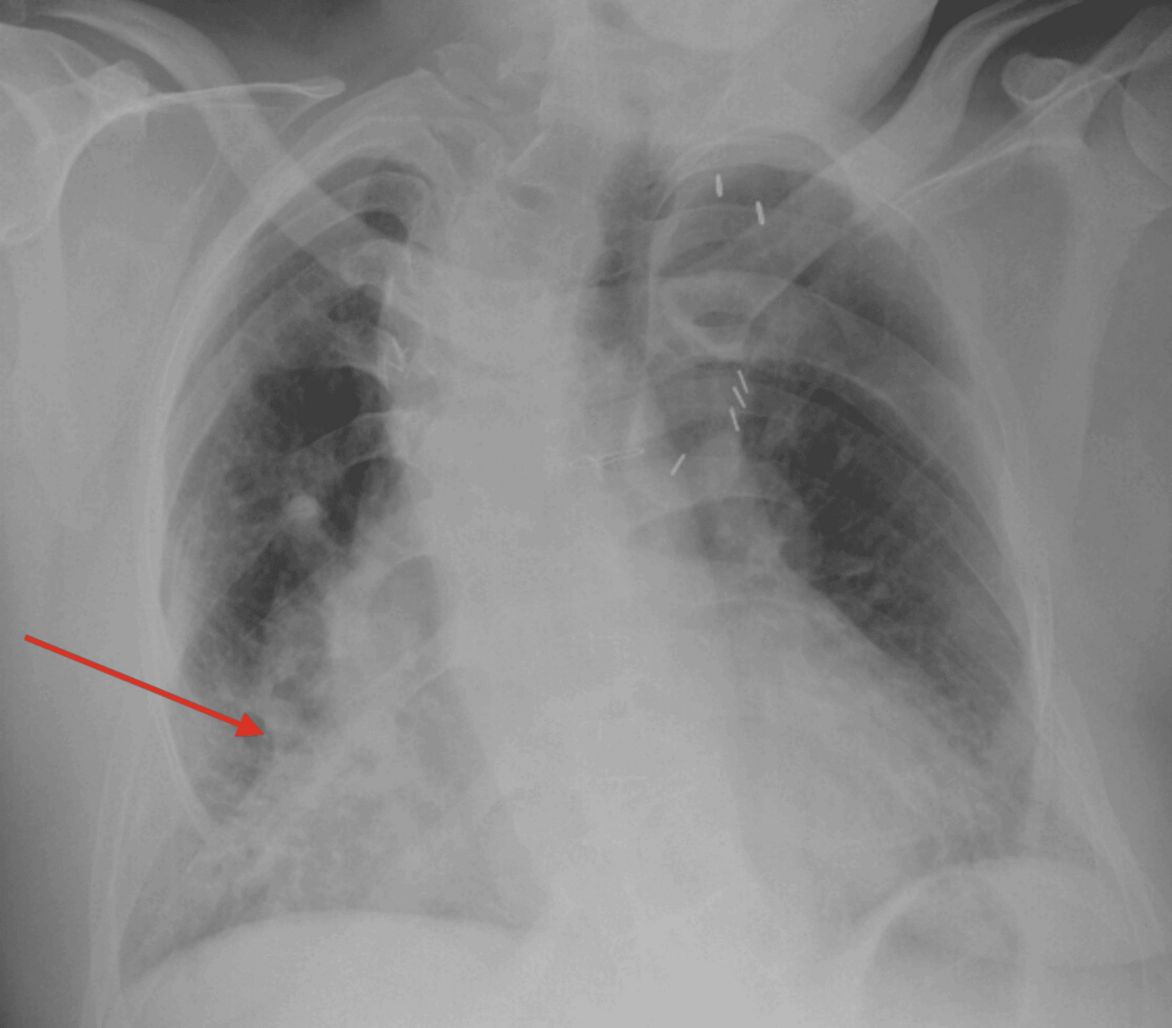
Initial X-ray demonstrating opacification of the right middle and right lower lobes
